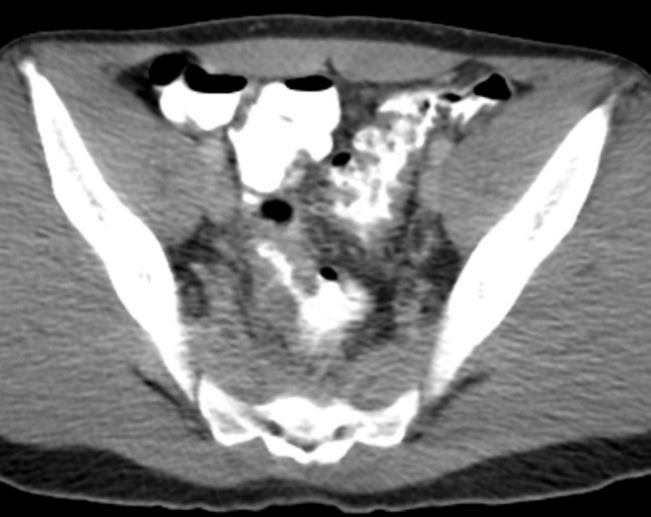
CT scan of the pelvis shows diffuse and “ulcer-like” severe thickening of the sigmoid wall with sparse diverticulas and perisigmoid fat infiltration.
